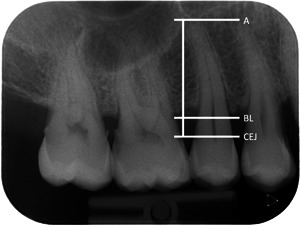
An example of RBL measurement in a periapical radiograph. RBL was calculated as the distance from the CEJ to the most coronal level of the alveolar crest (BL), where the periodontal ligament appeared with normal width. RBL was expressed as a percentage (%) of the total length of the root of the tooth, that is, CEJ to apex (A). BL, bone level; CEJ, cemento‐enamel junction; RBL, radiographic bone loss.
